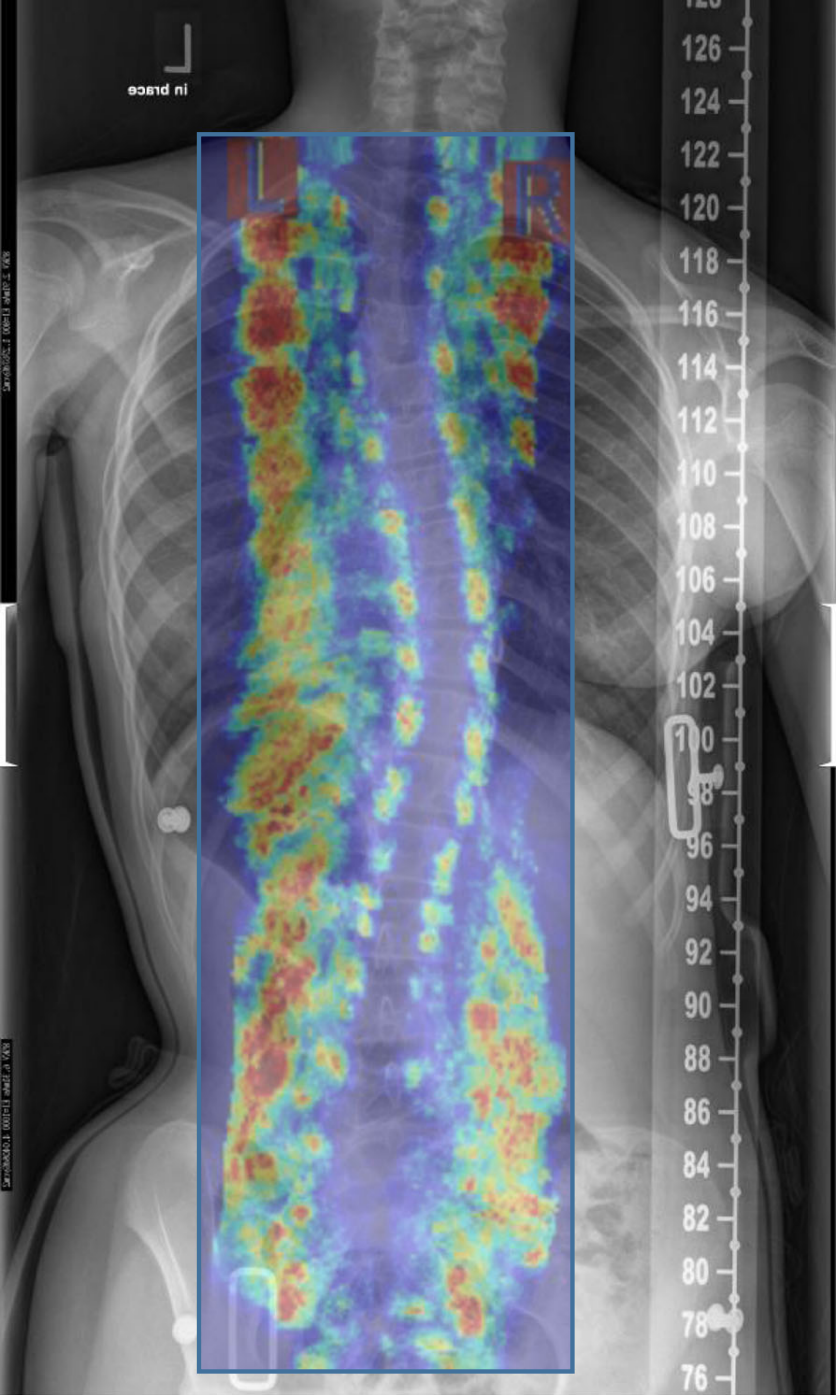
The second US trial overlapped with the in-brace radiograph in which the Cobb angle from the radiograph was 21°
